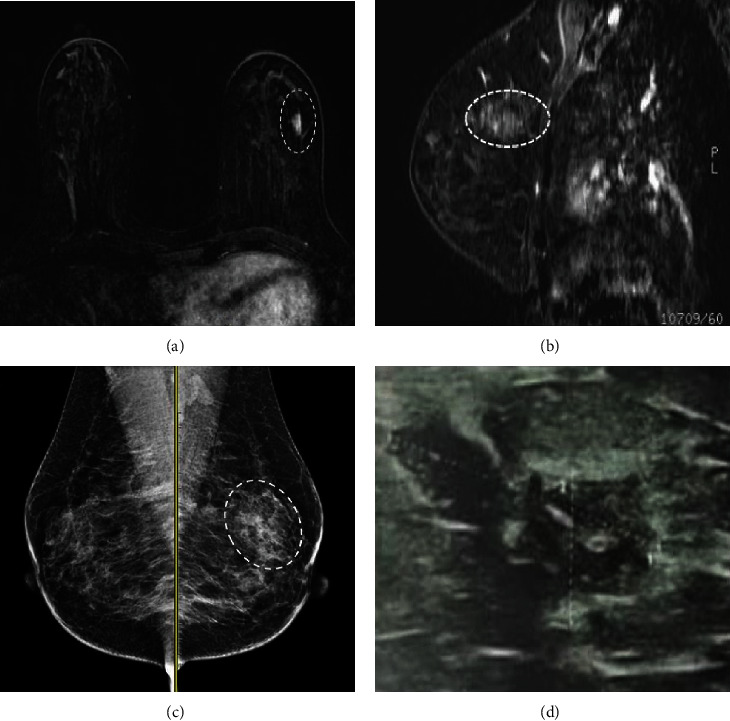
(a, b) Axial- and sagittal-subtracted contrast-enhanced breast MRI shows focal heterogenous NME in the upper outer aspect of the left breast (dashed circle). In MLO views (c), a focal asymmetry is seen (CC views are not shown) without microcalcification or architectural distortion. In targeted ultrasound (d), focal heterogenous fibroglandular tissue was identified. Pathology proved benign fibrocystic changes.
